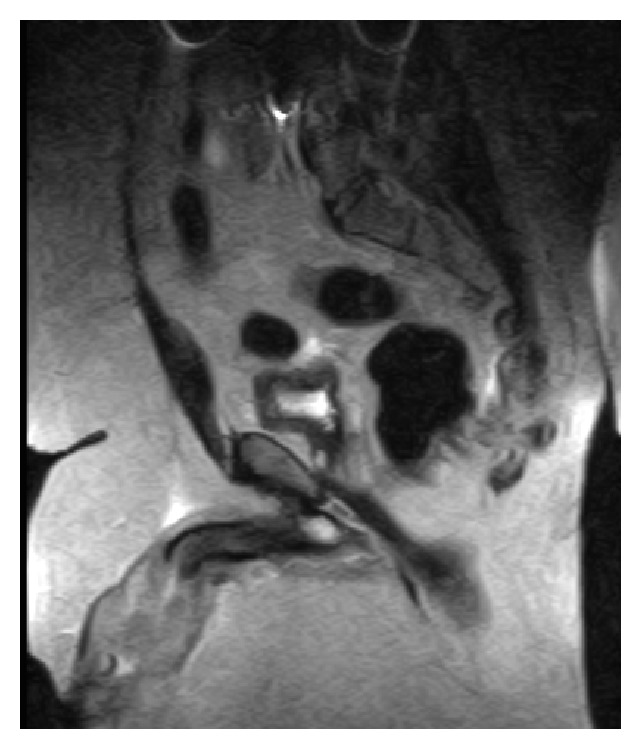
Coronal section of MRI showing the corporal bodies leading to a large mass buried within the suprapubic fat pad.
